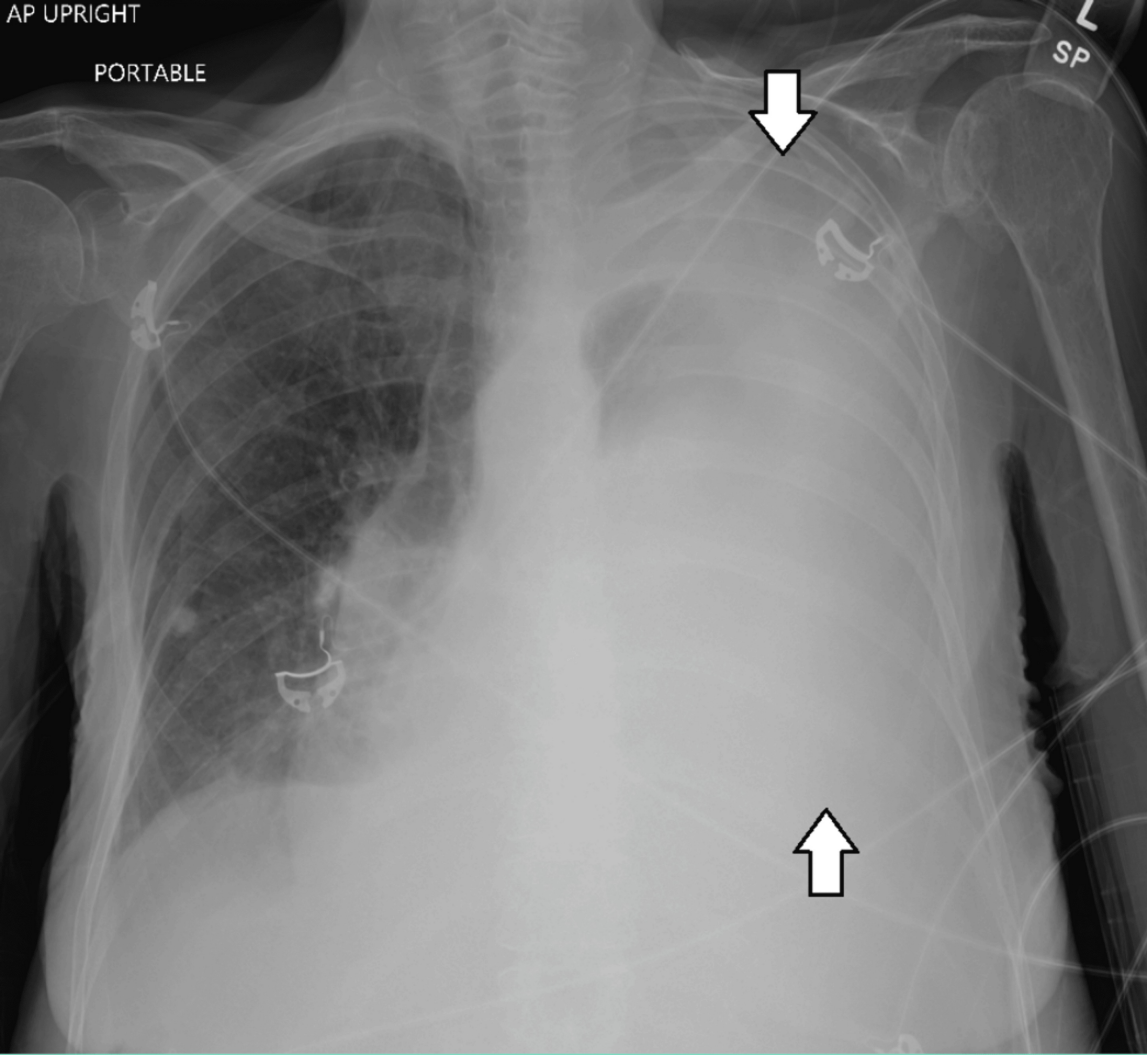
Portable anteroposterior chest X-ray view on arrival to the emergency department before thoracentesis.
